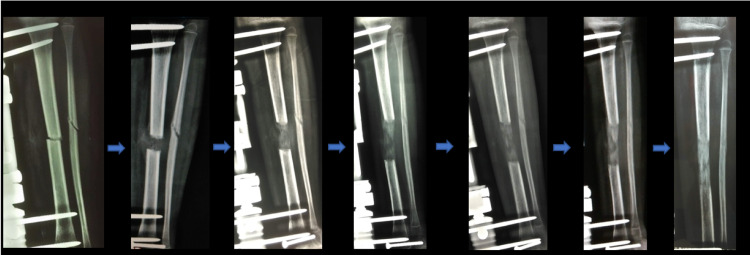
Osteotomy healing and bone lengthening progression of patient 2. From left to right, progressive X-ray images of patient 2 after osteotomy and application of the external fixator. This patient had the largest leg length discrepancy.
